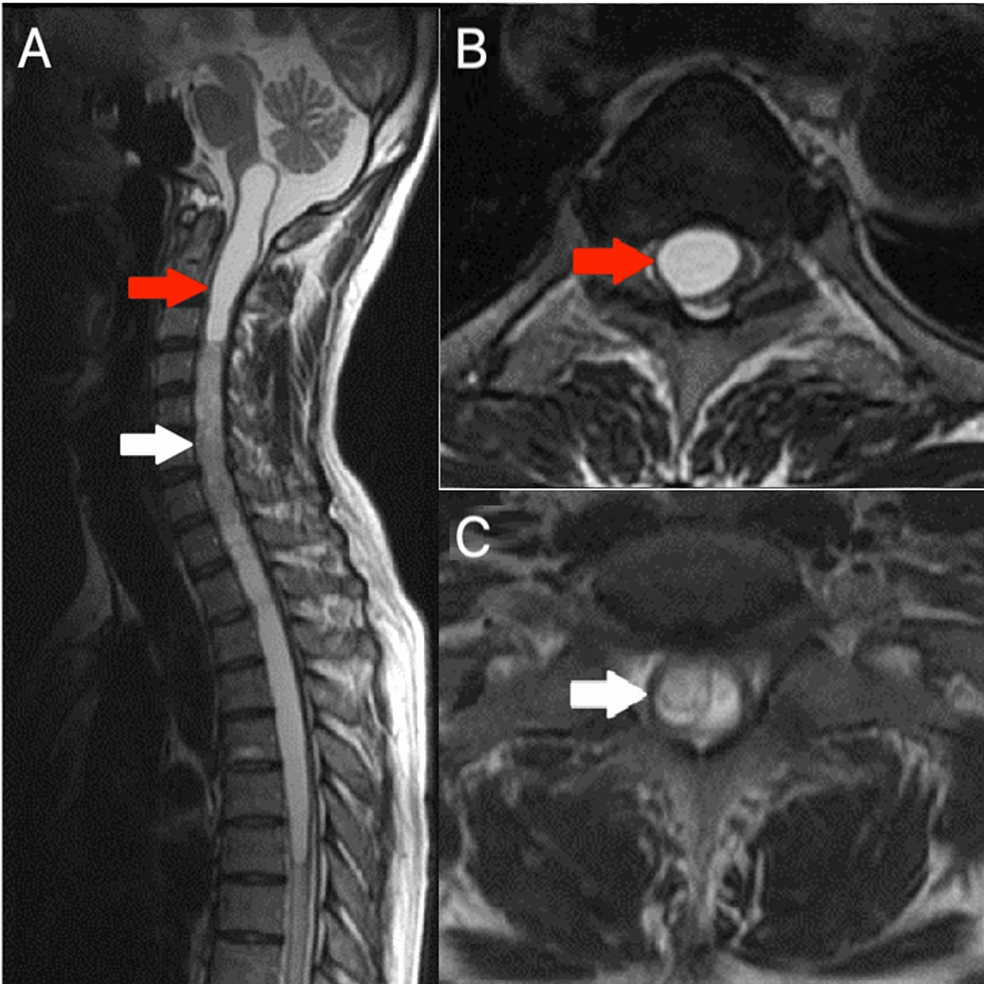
T2-weighted MRI in the sagittal plane (A) shows tumors from C4-C7 (white arrow) with syringomyelia upper and lower tumor (red arrow), corresponding to the axial plane through the syringomyelia (B) and through the tumor with multicystic components (C).
